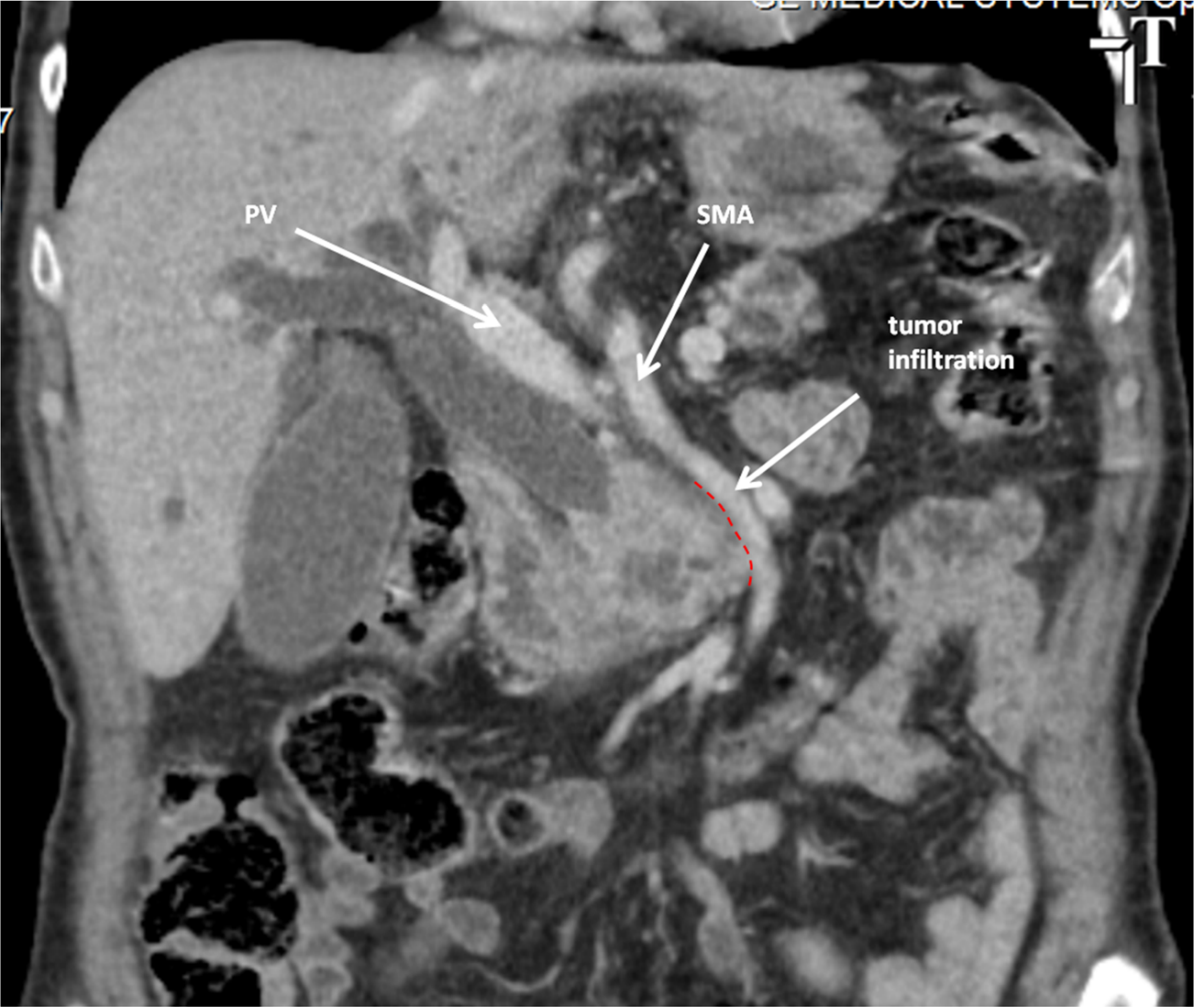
The preoperative CT-scan shows a 3-cm tumor infiltration of the superior mesenteric artery. *SMA* = superior mesenteric artery. *PV* = portal vein
